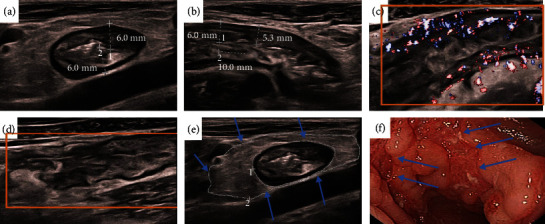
This male CD patient was 18 years old, and he had clinically active CD (CDAI = 360), which was characterized by abdominal pain, diarrhea, and wasting. In our examinations, this was a moderate-severe CD. The SES-CD and IBUS-SAS were 20 and 76 points, respectively. The serious segmental was the sigmoid colon. Application of the segmental activity and severity scores. (a, b) Bowel wall thickness (BWT): In longitudinal and cross-section orientations, BWT measurements were performed, and two measurement values were given in each orientation, [BWT] = [6.0 + 6.0 + 6.0 + 5.3]/4 = 5.8 mm. (c) Blood flow/color Doppler signal [CDS] = 3[short signals]. (d) Bowel wall stratification [BWS] = 3[extensive > 3 cm]. (e) Inflammatory fat [I‐fat] = 2[certain]. (f) Endoscopic image showing a longitudinal ulcer (arrow). The size of ulcer ≥ 4 cm. IBUS‐SAS = 5.8 × 4 + 2 × 15 + 3 × 7 + 3 × 4 = 76.
